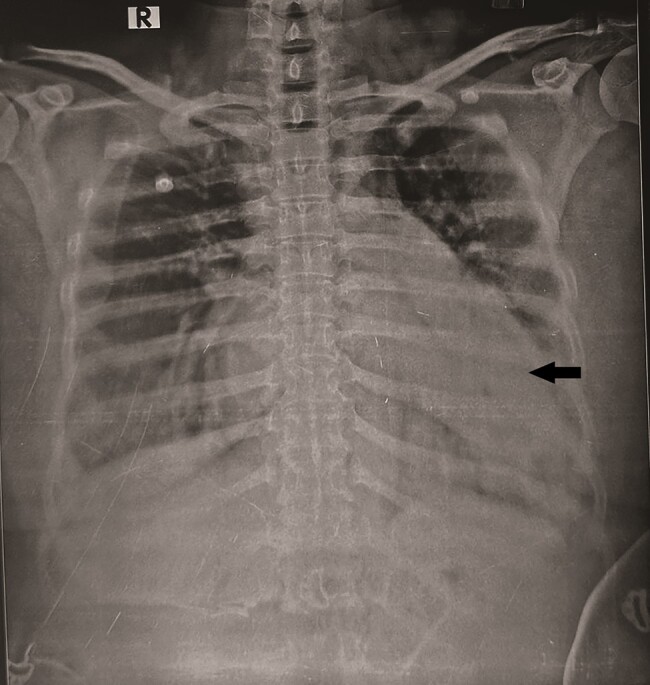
Chest X-ray showing mild para-hilar congestion and diffuse opacification in the left lower lobe of the lung (arrow).
